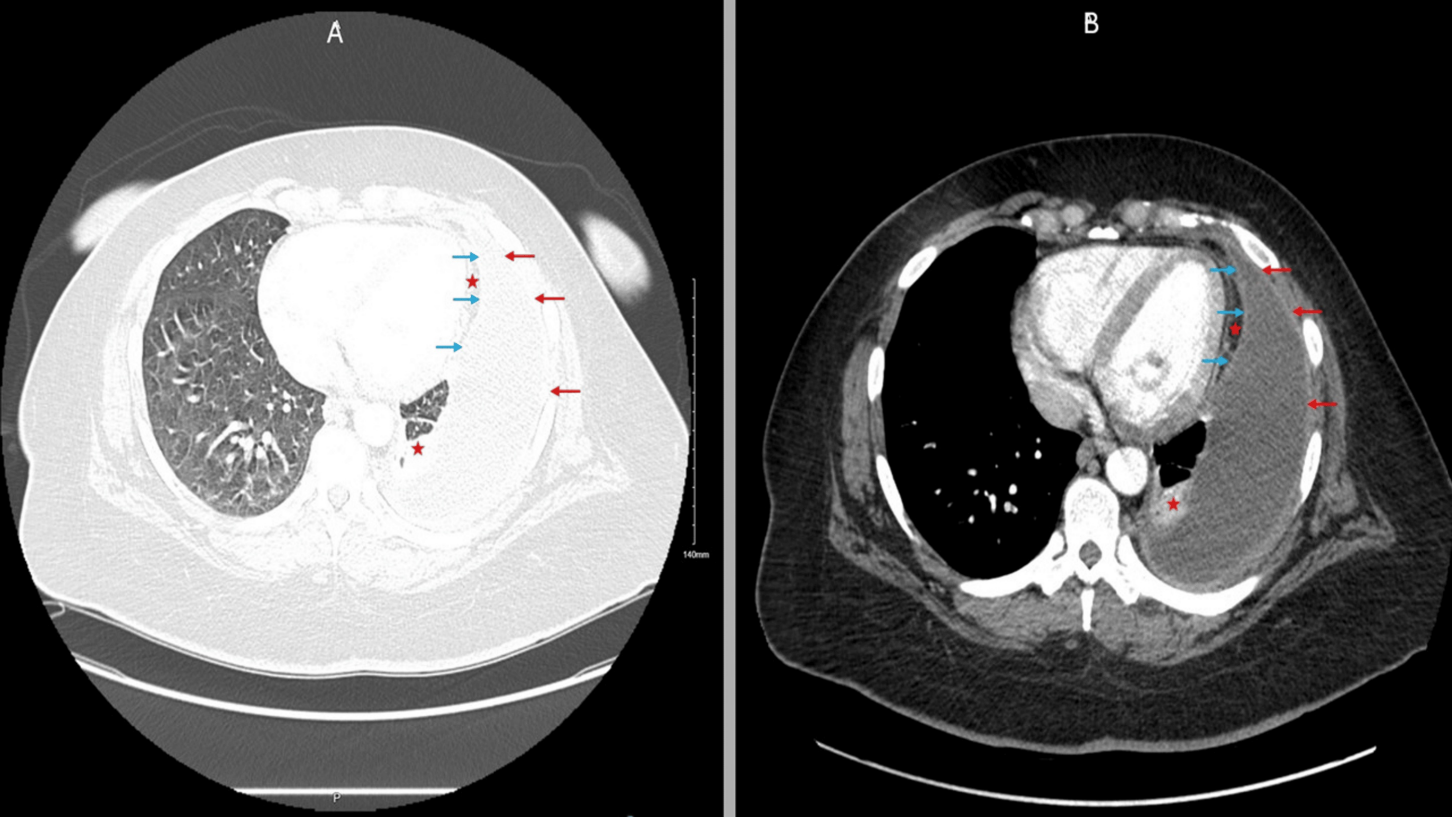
CT angiogram demonstrating split pleura sign in a large left pleural effusion (lung-window and standard views). No pulmonary embolism detected. Blue arrows: Thickened visceral pleura; Red arrows: Thickened parietal pleura; Red star: Compressive atelectasis. Panel A: Lung-window reconstruction. Panel B: Standard contrast-enhanced CTA view. Abbreviation: CTA = computed tomographic angiography.
